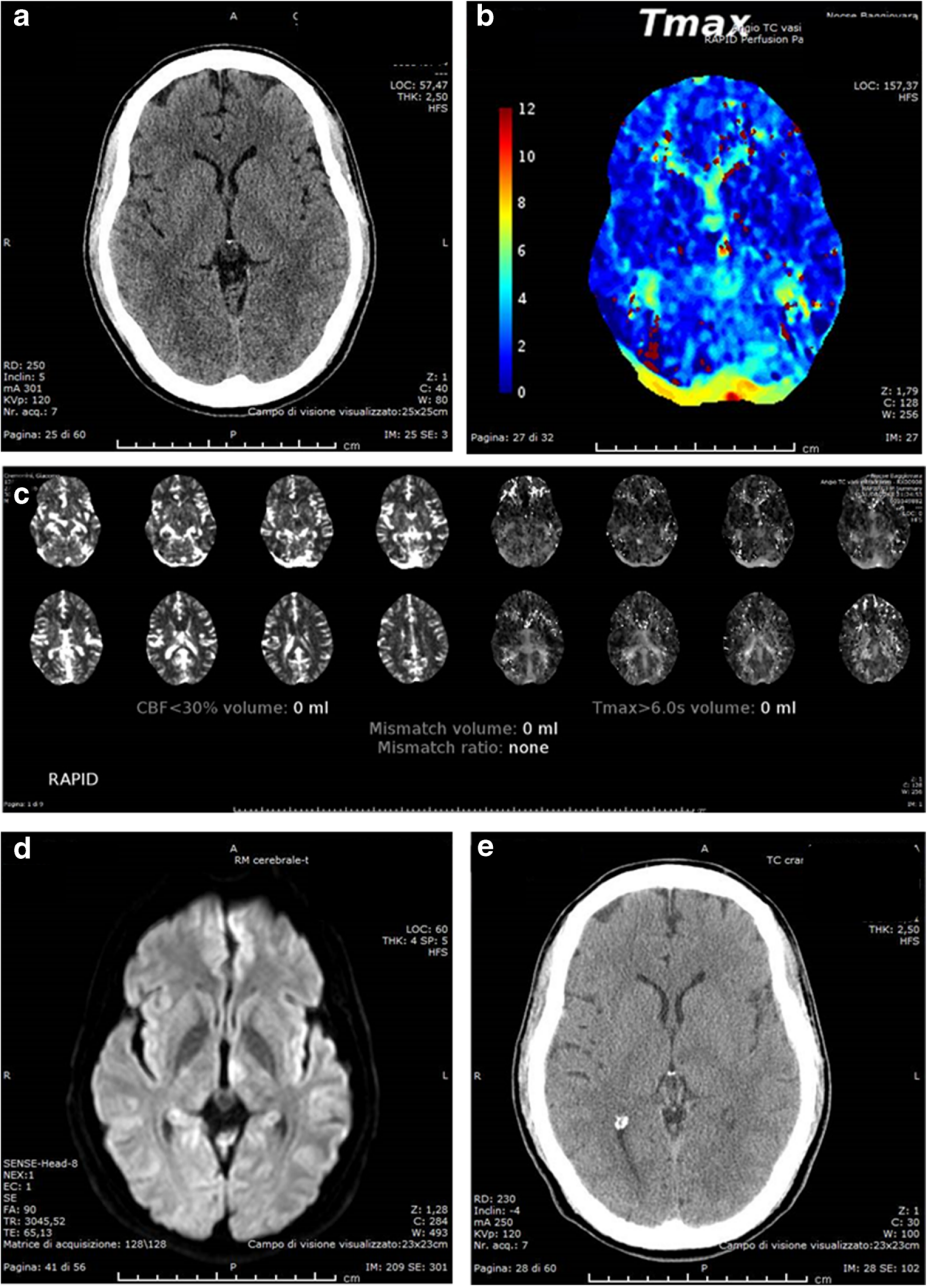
Left thalamic lacunar infarct: a ASPECTS 10 on initial NCCT, b RAPID colour-coded Tmax map shows perfusion alteration at left thalamic level but not displayed on RAPID automatic mismatch map (c); the lesion was confirmed on DWI (d) and also visible on follow-up CT (e)
